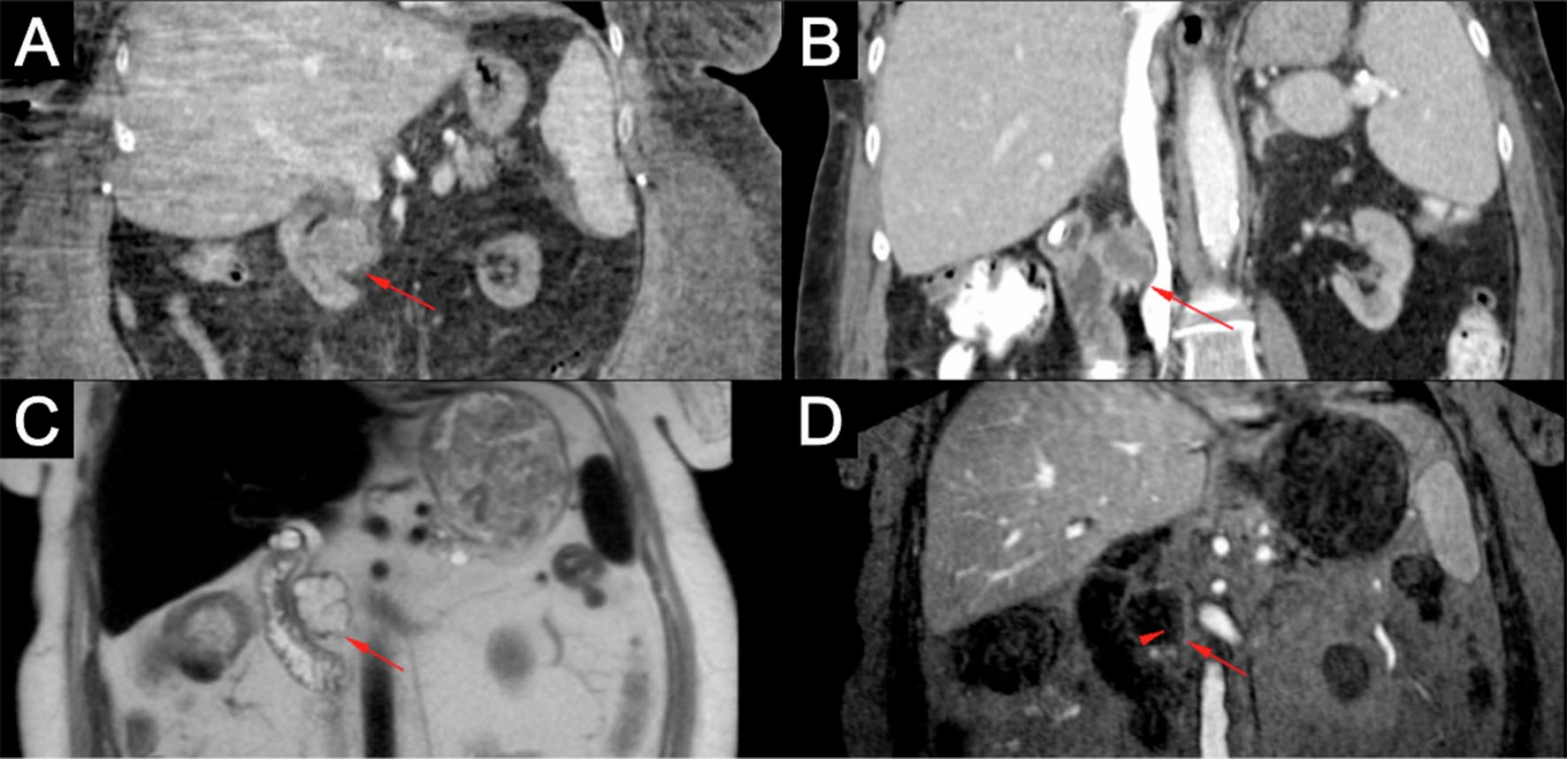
66 year-old female with a known stable cystic lesion in the pancreatic head. Contrast-enhanced CT 1 year prior to (a) and 2 years after (b) 1.5 T ferumoxytol-enhanced MRI (c, d) show a large cystic lesion (red arrows). Post-contrast T2 and T1 acquisitions show clustering of multiple cysts in the lesion (red arrows), thin septation (red arrowhead) and lack of suspicious enhancing components suggestive of a serous cystadenoma or intraductal papillary mucinous neoplasm
